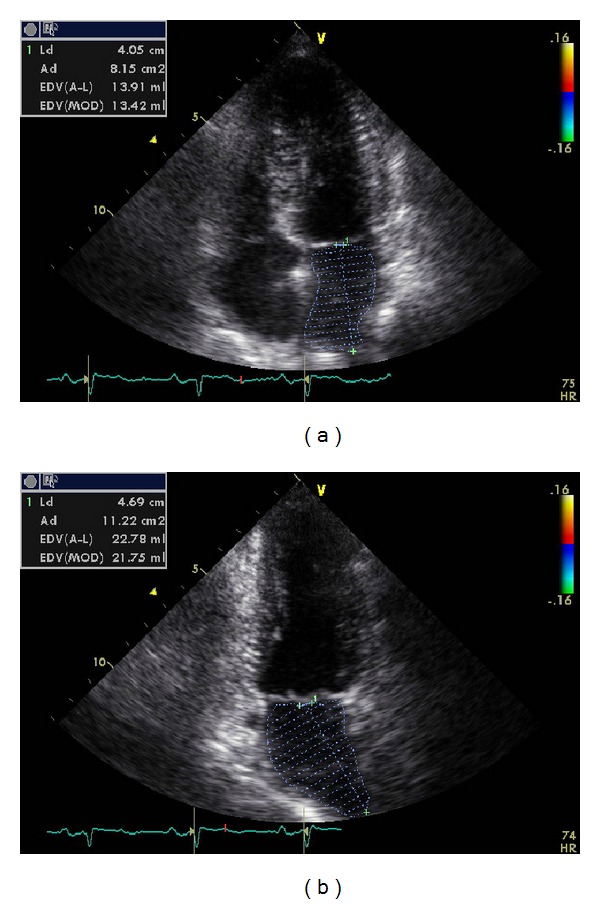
Measurement of left atrial volume from biplane method of disks (modified Simpson's rule) using apical 4-chamber (a) and apical 2-chamber (b) views at ventricular end-systole (maximum volume).
